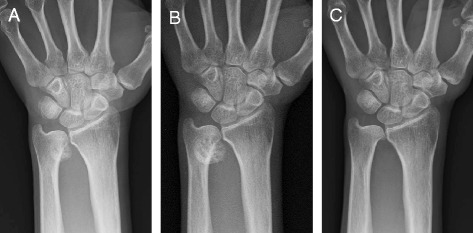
AP radiographic findings. a The first AP radiograph shows calcifications within the distal radioulnar joint (DRUJ). b The preoperative AP radiograph shows a bony prominence arising from the distal end of the ulna. c At follow-up 2 years after surgery. The AP radiograph indicates that there has been no recurrence of the lesion
